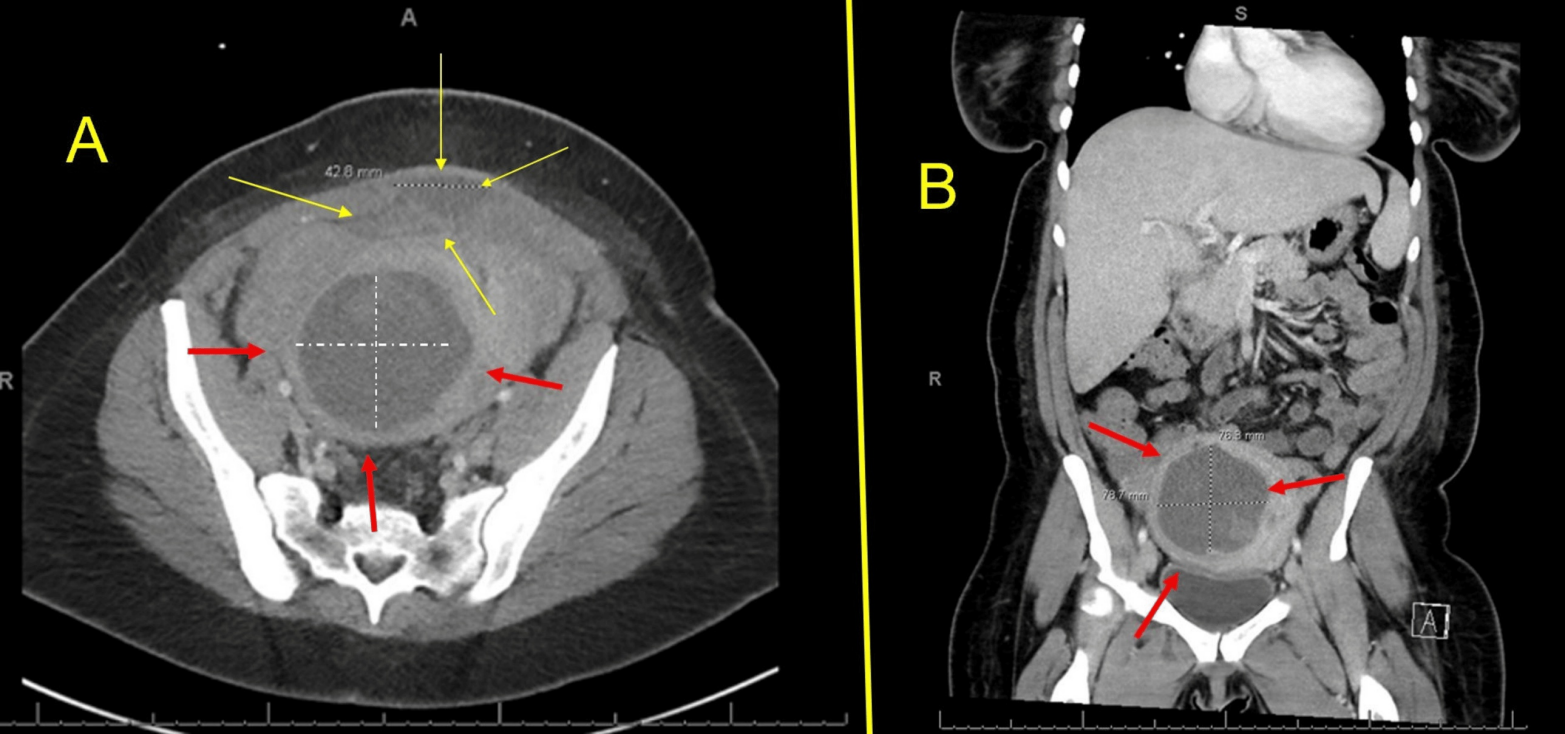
CT scans of the pelvis (transverse, image A) and abdominal pelvic (coronal, image B) (day 50 after initial admission and 35 days after cesarean delivery). The fluid collection in the uterus had decreased slightly to 7.6 cm x 7.9 cm (between hash marks in images A and B), and differential diagnosis included degenerated necrotic uterine fibroid versus myometrial abscess. Concern for the progression of an abscess in the anterior pelvic wall with an increase in all dimensions, measuring now 4.2 cm (long yellow arrows in image A). The red arrows in images A and B are the uterine wall.
